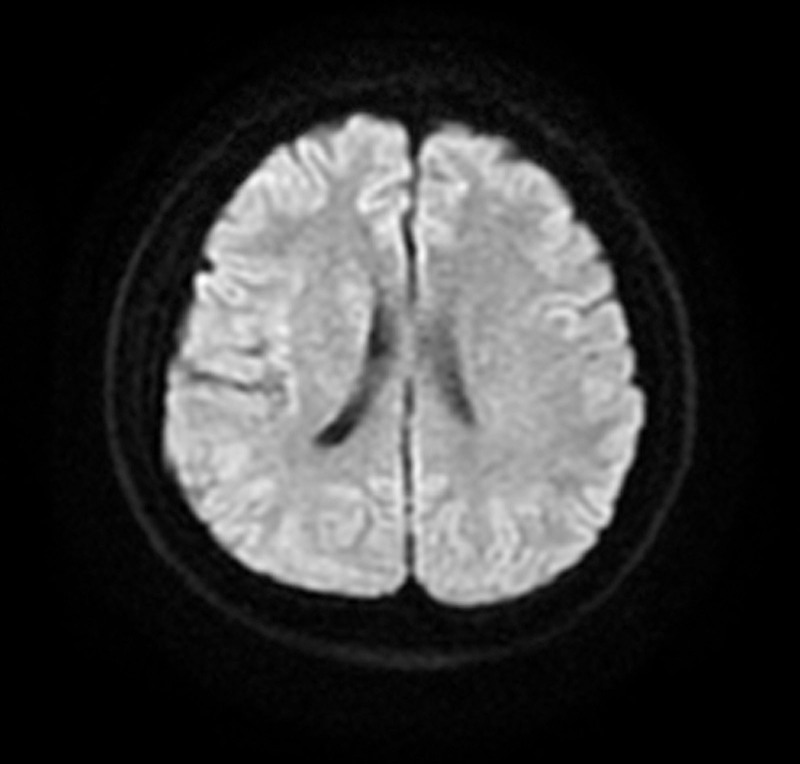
No cerebral infarcts were found using magnetic resonance imaging.
